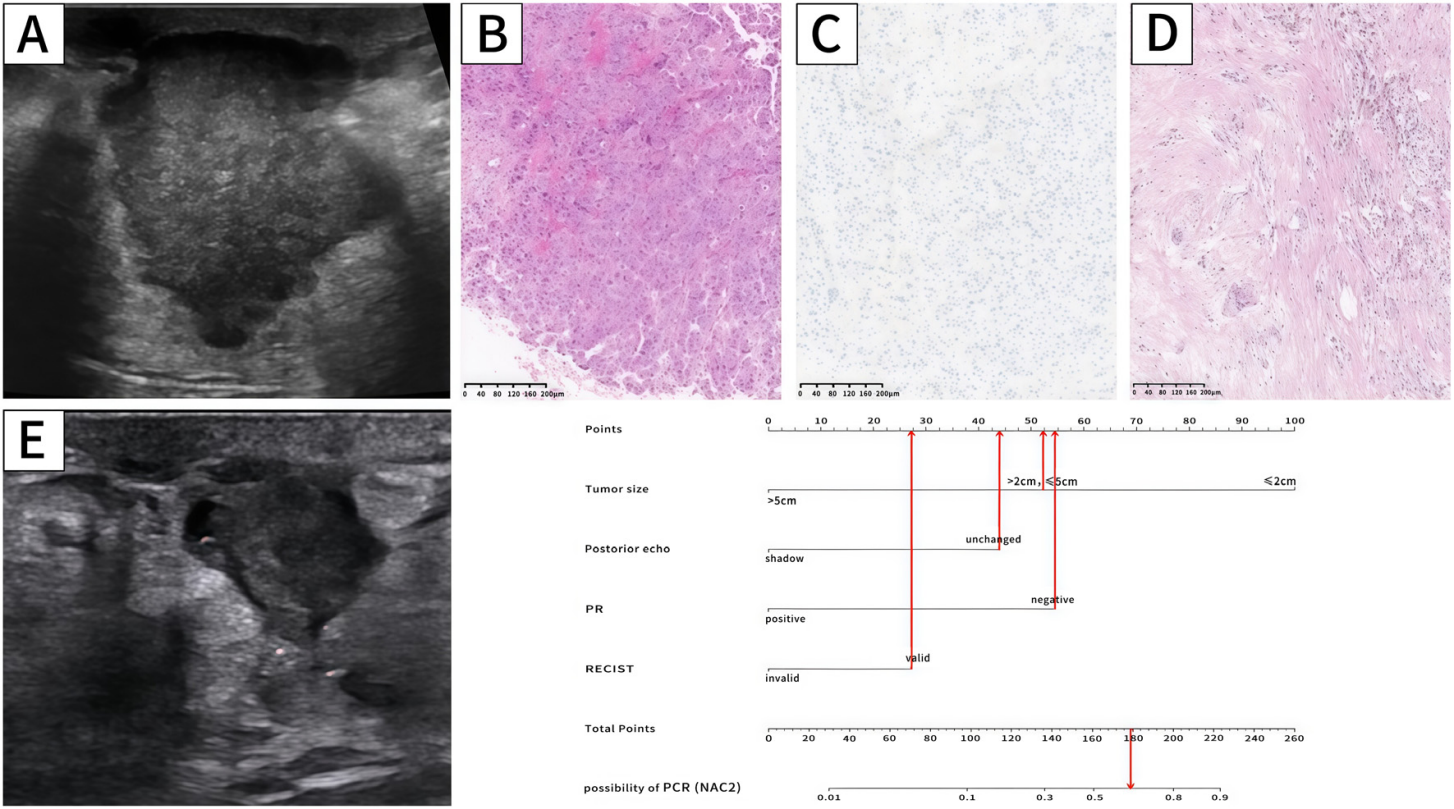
Ultrasound,pathology and nomogram of a breast cancer patient. Ultrasound before NAC (A) revealed that the maximum tumor diameter was 3.2cm (52.5 points),and there was no posterior shadow (44 points). Biopsy pathology (B) confirmed breast cancer,and IHC (C) showed PR (-) (54.5 points).Ultrasound after NAC2 (E) showed that the maximum tumor diameter reduced to 1.6cm, and the RECIST was valid (28 points). The total score is 179 (52.5 + 44+54.5+28 = 179 points). The probability of this patient achieving PCR was approximately 0.64. The pathology after mastectomy (D) showed MP5 and the patient achieved pCR.
